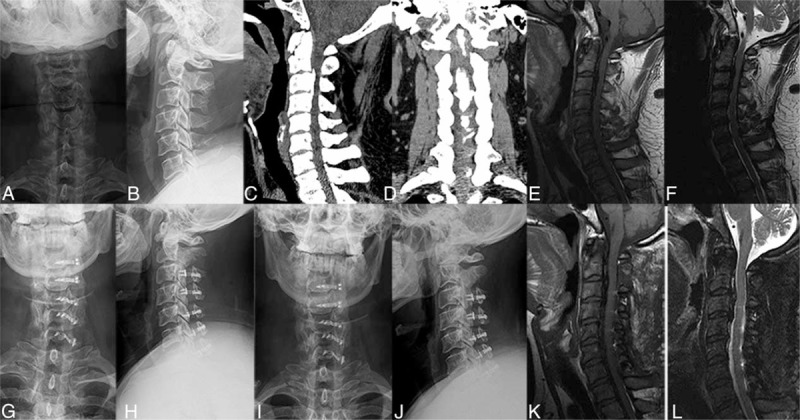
OPLL: A 37-year-old male patient (A-L), preoperative X-ray (A, B), CT (C, D), MRI (E, F), 1 week (G, H), and 1 year (I, J) after operation the X-ray showed good internal fixation; 1 year after operation the MRI examination (K, L) showed spinal cord without compression. OPLL =  ossification of posterior longitudinal ligament.
